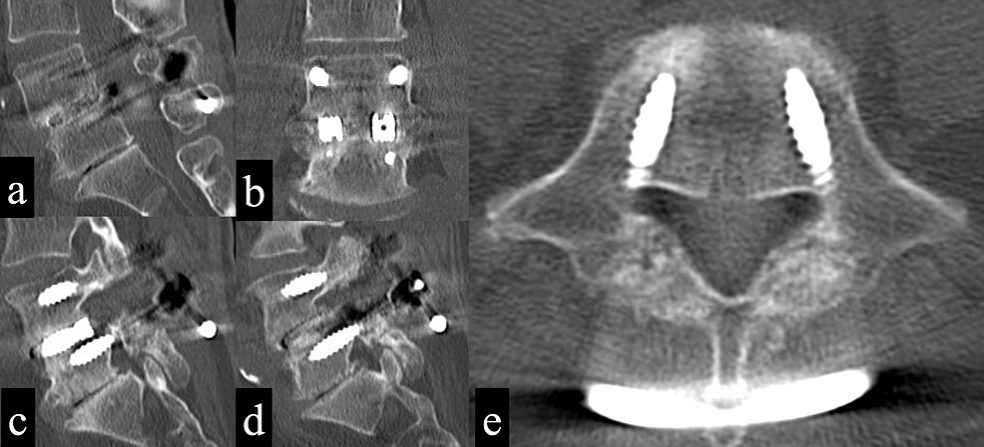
Imaging findings of three months after surgery 3a: A plain CT sagittal view (midline) shows bony bridging between L4/5. 3b: A plain CT coronal view shows bony bridging between L4/5. 3c: A plain CT sagittal view (right) shows bony fusion at the L5 right pars cleft. 3d: A plain CT sagittal view (left) shows bony fusion of the L5 left pars cleft. 3e: A plain CT axial view showing bony fusion at the L5 bilateral pars clefts.
